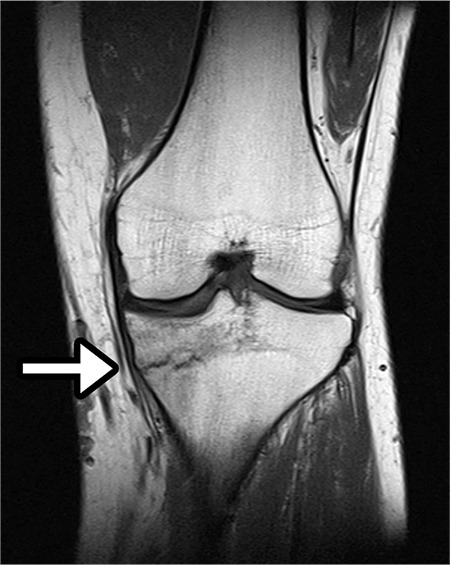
Magnetic resonance image confirming the stress fracture line in the proximal tibia.
